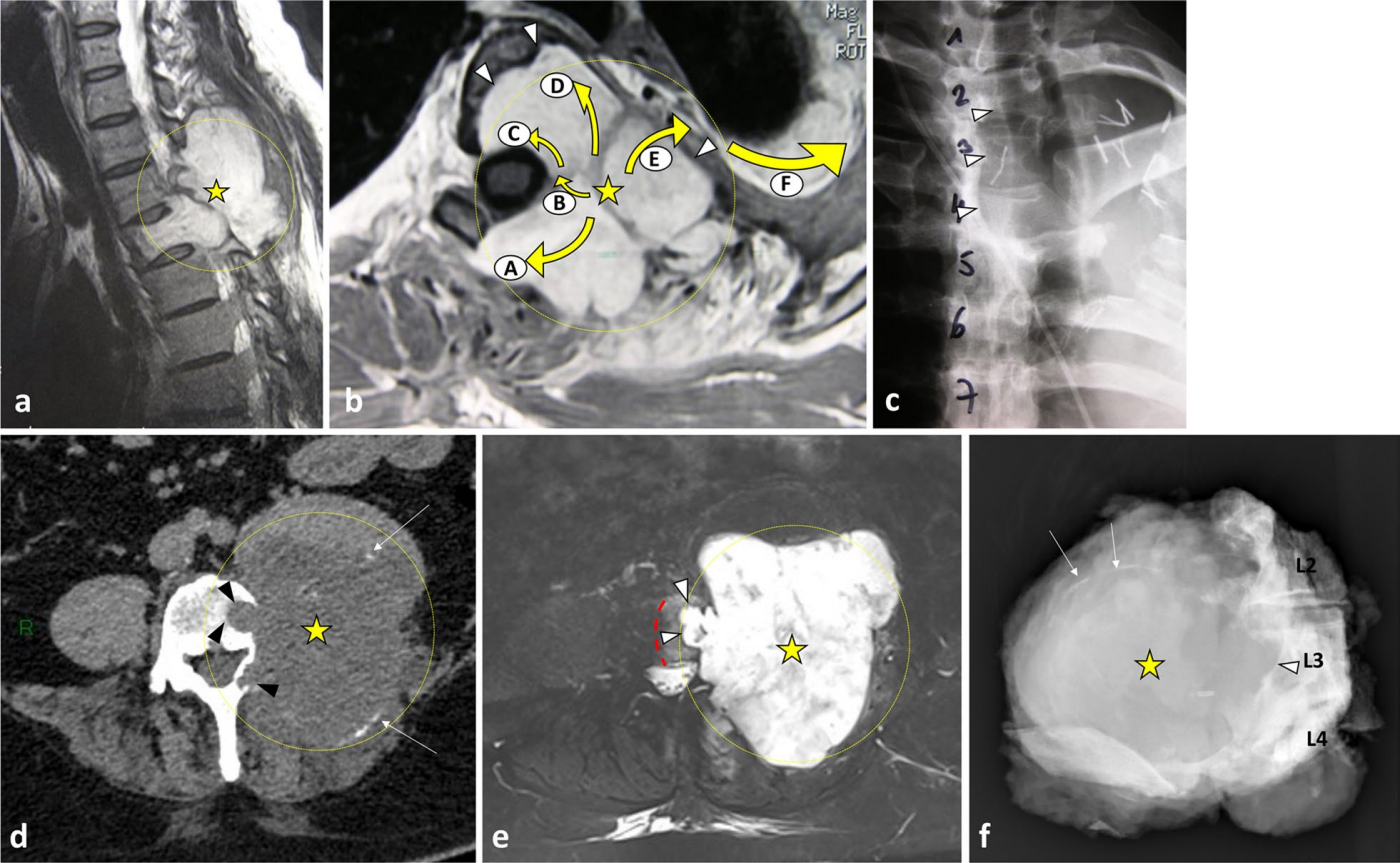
Origin and extent of the paraspinal tumours. Myoepithelial soft tissue tumour* of paraspinal muscles in a 52-year-old male (with acute thoracic back pain) that secondary invades the 3rd thoracic vertebra and the 2nd, the 3rd and the 4th ribs (a, b, c). Sagittal T2WI (a) and axial T1WI with contrast agent (b) show a paraspinal tumour with an epicentre in epaxial muscles (star). Arrows show tumour’s spread towards the opposite side (A), into the bone (D), into the epidural space (C) via the intervertebral foramen (B), in the pleura (F) via the intercostal space (F). Postoperative anteroposterior radiograph shows a lysis of the 3rd and 4th vertebras (white arrowheads). The ribs were removed. *Chondrosarcoma* of the pedicle-transverse part of L3, that secondary invades the hypaxial and epaxial muscles, in a 62-year-old male (d, e). Axial CT reconstruction (d) and axial T2WI (e) show a mass with a cartilage matrix including calcifications (white arrows, (d)) and high T2WI intensity (e). The mass presents aggressive bone lysis (arrowheads) surrounded by inflammation (red dashed line). Posteroanterior radiograph of the surgical sample of vertebrectomy and pelvic resection (f) shows a shadow and calcification (arrows) and lysis of the vertebral body and transverse process of L3 (arrowhead)
